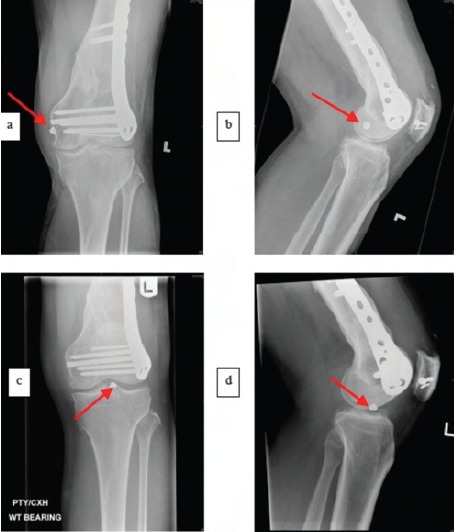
Radiographs with red arrows indicating the migrated
